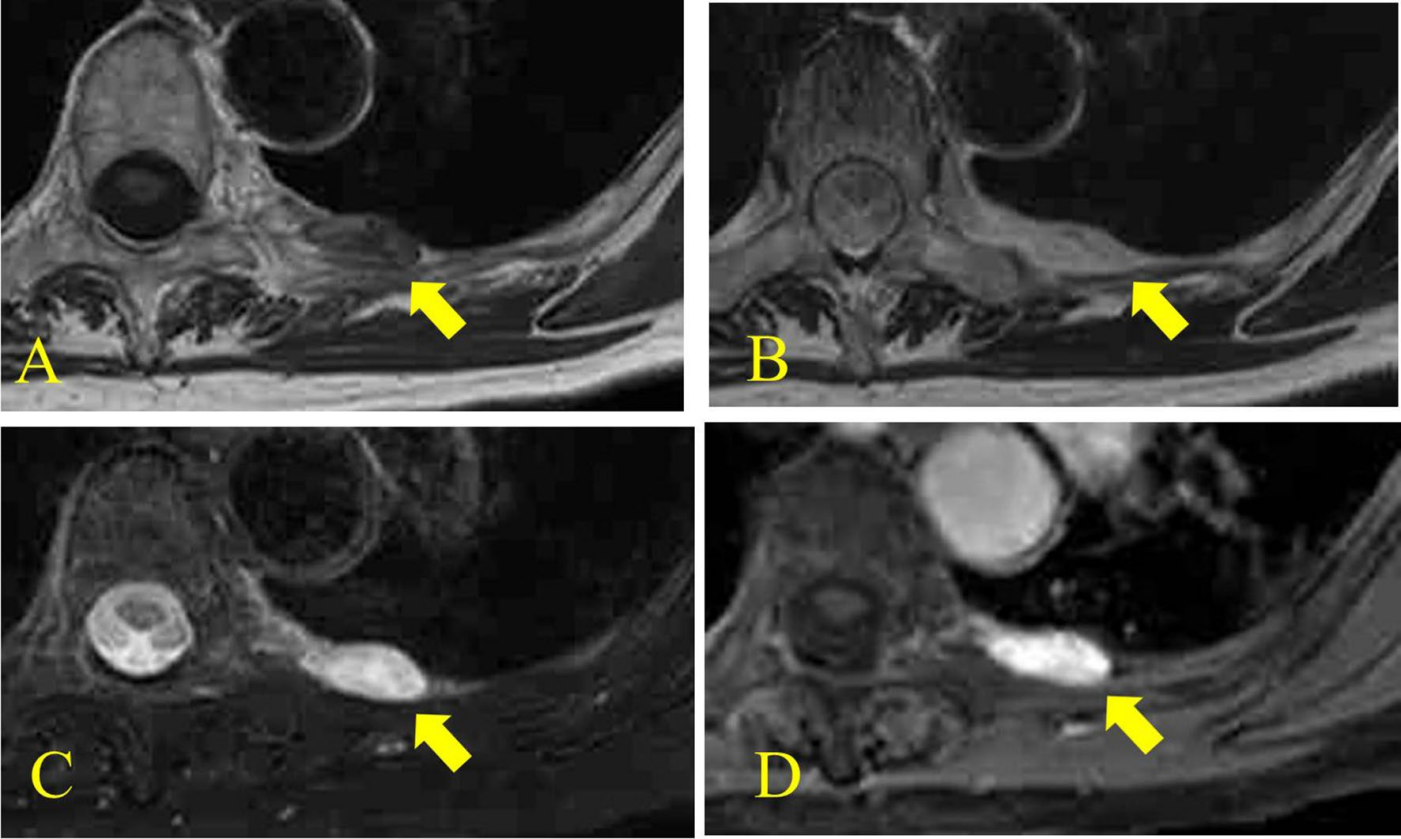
Chest MRI findings. The lesion showed low signal intensity on T1-weighted images (A), heterogeneous high signal intensity on T2-weighted images (B) and high signal intensity on fat-suppressed T2-weighted images (C). The lesion showed heterogeneous enhanced effect on gadolinium-based contrast agent (D)
